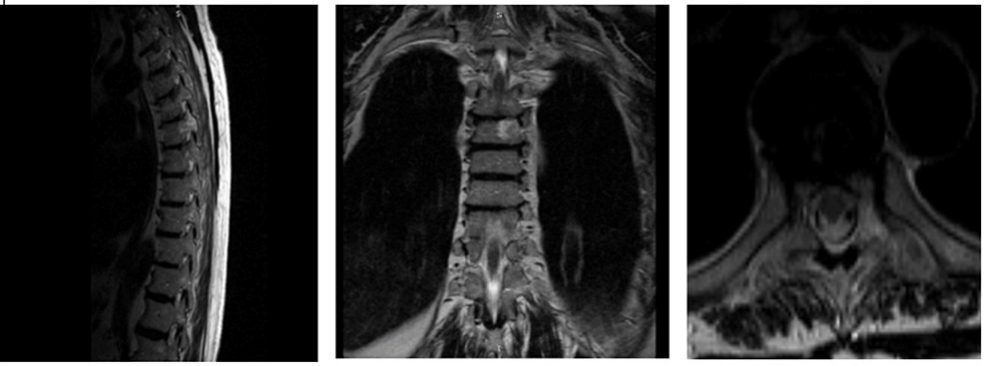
Preoperative surgical stage, from left to right: (A) sagittal, (B) coronal, and (C) axial thoracic magnetic resonance imaging slides on T2. The images show osteochondritis of the thoracic spine, scoliosis on the right side, hemangioma of the Th6 body of the vertebra, and Forestier’s disease.
